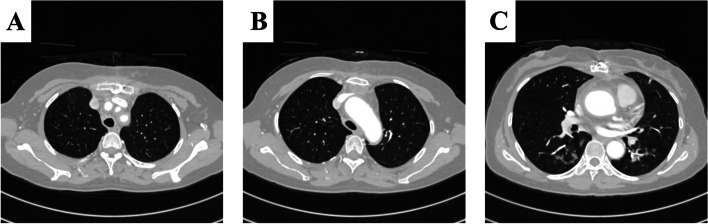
High-resolution chest CT images showing primary outcomes following therapeutic fiberoptic bronchoscopy. Panels A-C display trachea, bronchus, and basal bronchus, respectively (indicated by red arrows), while the great vessels are highlighted by the yellow arrow
